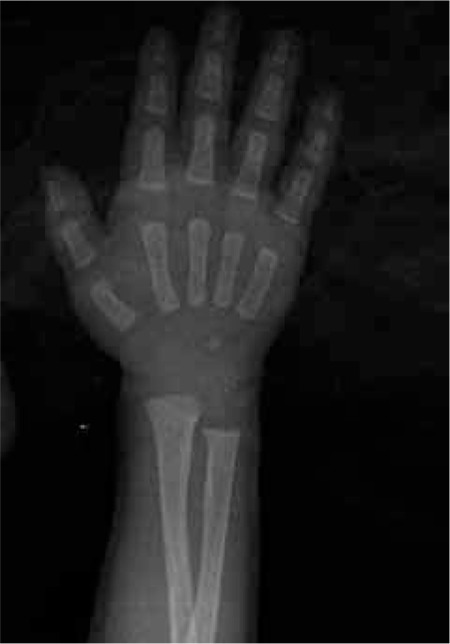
Case 1- improvement of the metaphyseal irregularity on wrist X-ray
